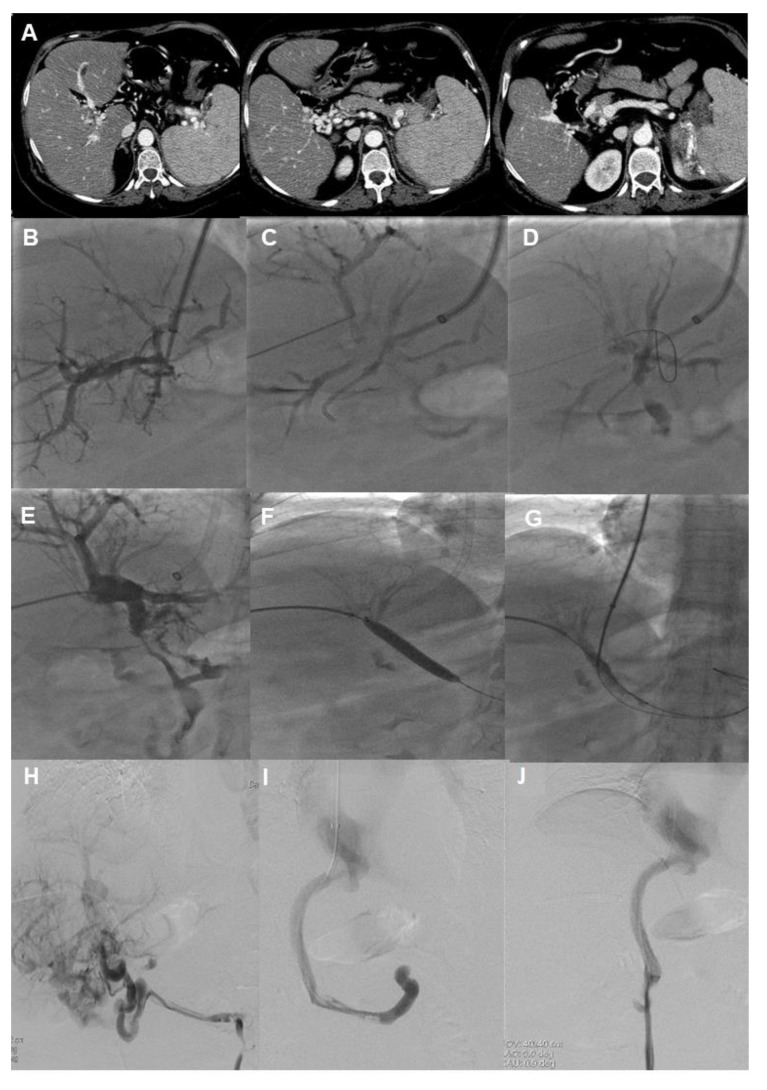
Example of a patient with idiopathic nonmalignant noncirrhotic portal vein cavernoma. The patient was admitted for recurrent upper gastrointestinal bleeding episodes from a duodenal varix, thus the creation of an elective TIPS for secondary prophylaxis of rebleeding was attempted. (A) Abdominal CT scan showing the portal cavernoma and patent spleno-mesenteric confluence. (B) The Colapinto needle was advanced from the right hepatic vein into a portal vein radicle using real-time sonographic and fluoroscopic guidance. However, it was not possible to catheterize the calcified portal vein remnant. (C,D) US-guided percutaneous puncture and subsequent catheterization of a portal vein radicle were performed with an intercostal approach. (E) A 6F introducer system was advanced into the intrahepatic portal vein system util the origin of the calcified portal vein remnant. (F) A hydrophilic wire was successfully introduced through the remnant and into the spleno-mesenteric confluence. Subsequent dilatation of the portal vein remnant was performed with 6 mm, 8 mm, and 10 mm balloon catheters. (G) The ballon was used as a landmark for portal vein puncture with a Colapinto needle, and subsequently, portal system catheterization was performed. (H) Portography performed before and (I) after TIPS creation with a Viatorr 10 mm diameter e-PTFE-covered stent. (J) Of note, there is no filling of varices at the final portography.
